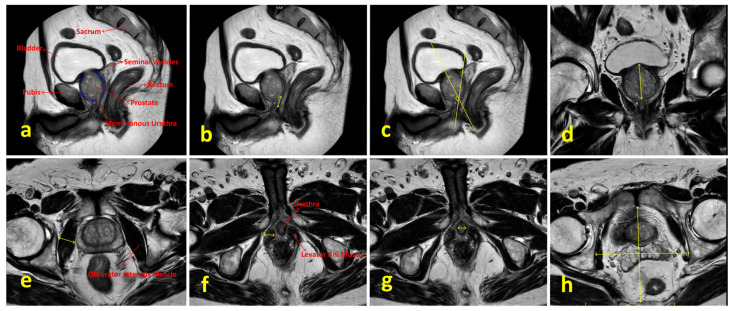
Multiparametric MRI parameters of the patients and the methods used for their measurement. (a) Sagittal T2-weighted image showing relevant pelvic anatomical structures. (b) Measurement of membranous urethral length. (c) Measurement of membranous urethra–prostate axis angle. (d) Measurement of intraprostatic urethral length in the coronal plane. (e) Measurement of obturator internus muscle thickness. (f) Visualization of the prostate apex, membranous urethra and levator ani muscle; levator ani muscle thickness is indicated by yellow arrows. (g) Measurement of urethral width. (h) Measurement of pelvic anterior–posterior and transverse diameters.
